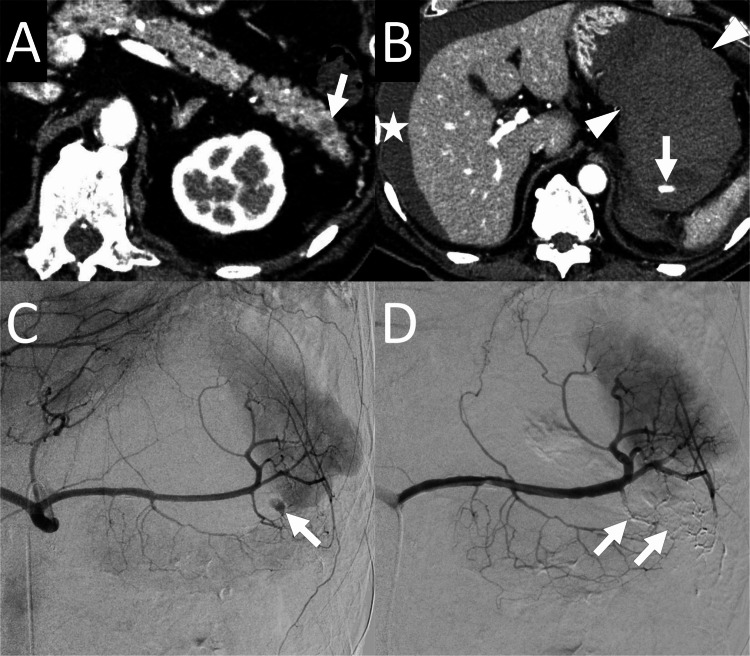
74-year-old man who underwent transcatheter arterial embolization for bleeding caused by endoscopic ultrasound-guided fine-needle aspiration (EUS-FNA) for a pancreatic tail lesion (patient number 4) A: An axial arterial-phase contrast-enhanced computed tomography (CECT) image obtained before EUS-FNA reveals a hypodense lesion (arrow) in the pancreatic tail. B: An axial arterial-phase CECT obtained after EUS-FNA reveals contrast-media extravasation (arrow) near the splenic hilum. A large hematoma (arrowheads) surrounding the extravasation and bloody ascites (star) are observed. C: Angiography of the celiac artery reveals a pseudoaneurysm (arrow) in the caudal pancreatic artery. D: Angiography of the splenic artery after embolization of the caudal pancreatic artery with N-butyl cyanoacrylate (NBCA) reveals no opacification of the pseudoaneurysm. NBCA cast (arrows) is observed in the caudal pancreatic artery.
